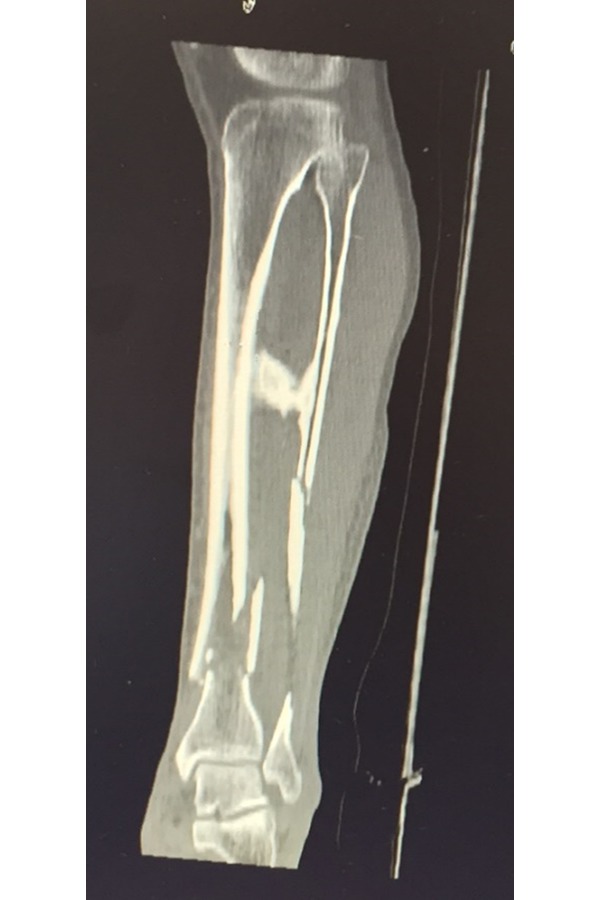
CT scan left leg confirms leg fracture and synostosis
